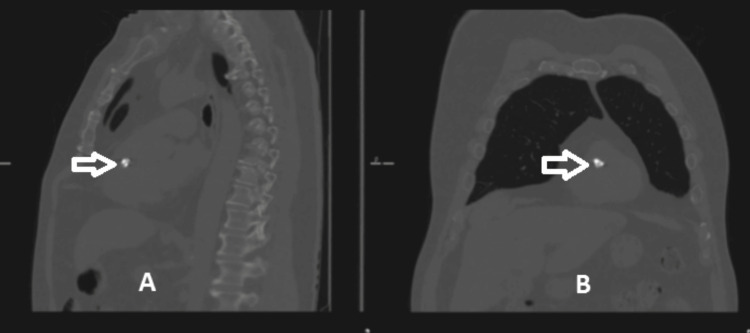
(A) A sagittal cut of the CT chest, with an arrow pointing to the dense mass; (B) a coronal cut of the CT chest, with an arrow pointing to the dense mass. CT, computed tomography
